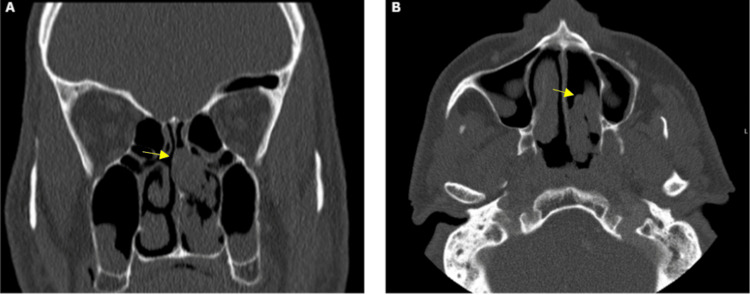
Computed tomography of the paranasal sinuses, coronal (A) and axial (B) views show a grade 4 polyp in the superior part of the left nasal cavity compressing the left middle turbinate and obliterating the left sphenoethmoidal recess as indicated by arrows
